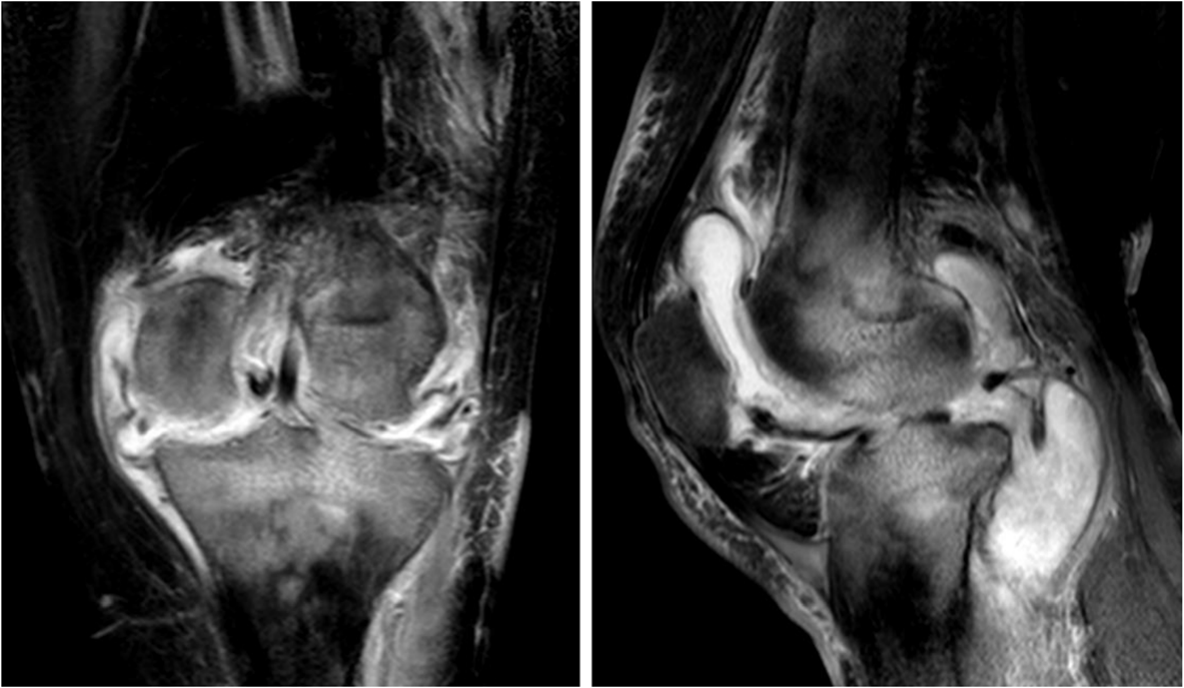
MRI (anteroposterior and lateral): synovial hypertrophy, unclear boundary of surrounding soft tissue, clear scope of cold abscess, severe joint destruction, obscure subcartilaginous bone plates, and central and peripheral erosions
